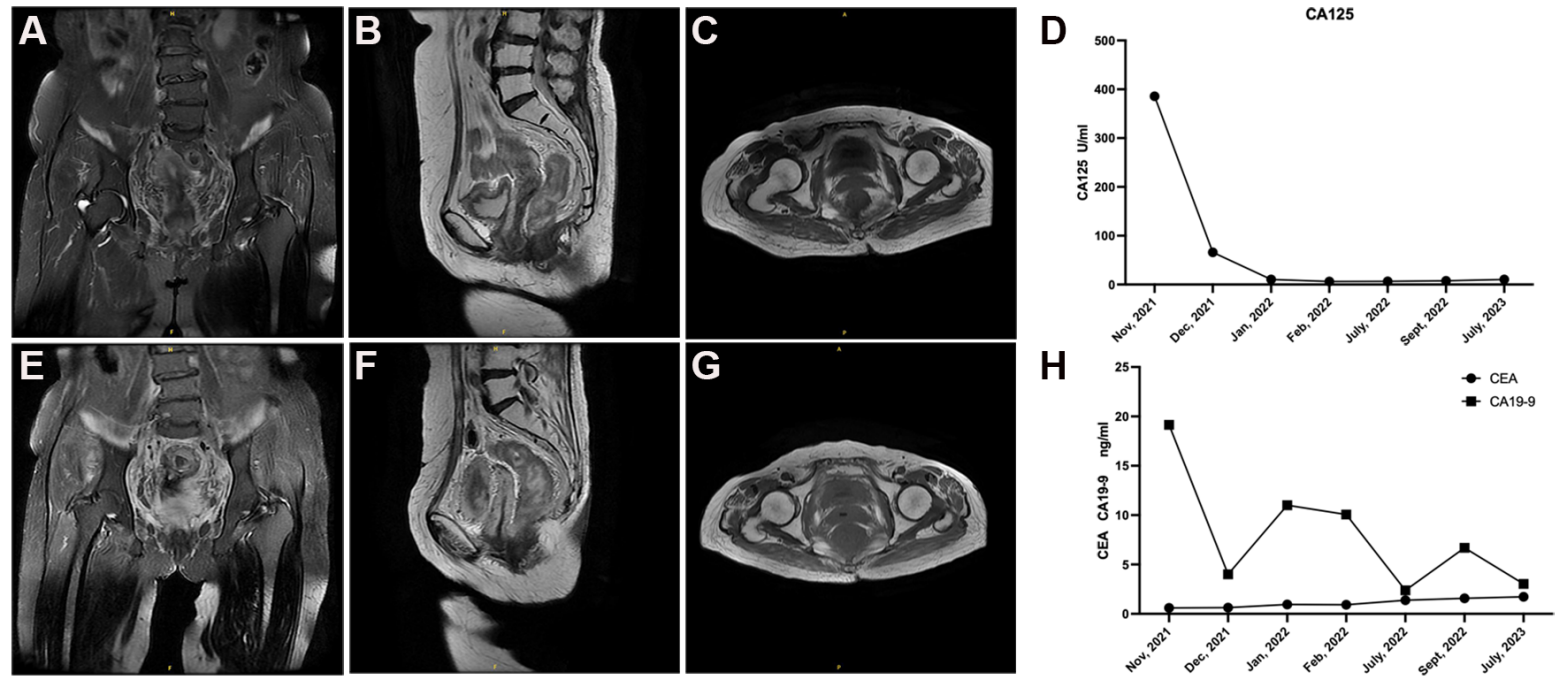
Follow-up pelvic MRI and tumor marker results in the patient with G-EAC. (A–C) The patient exhibited a substantial reduction in lesion size on MRI following radiotherapy and chemotherapy. The maximum cross-sectional dimension was measured to be approximately 1.3 cm×2.0 cm. (E–G) Regular pelvic MRI follow-up examinations revealed no signs of tumor recurrence in the postoperative period for this patient. (D) Prominent reduction in CA125 levels observed in the patient's blood after commencement of chemotherapy, demonstrating sustained lower levels throughout subsequent follow-up period. (H) CA19-9 and CEA levels fluctuated within the normal range, accompanied by a remarkable decrease in CA19-9 subsequent to radiotherapy and chemotherapy.
